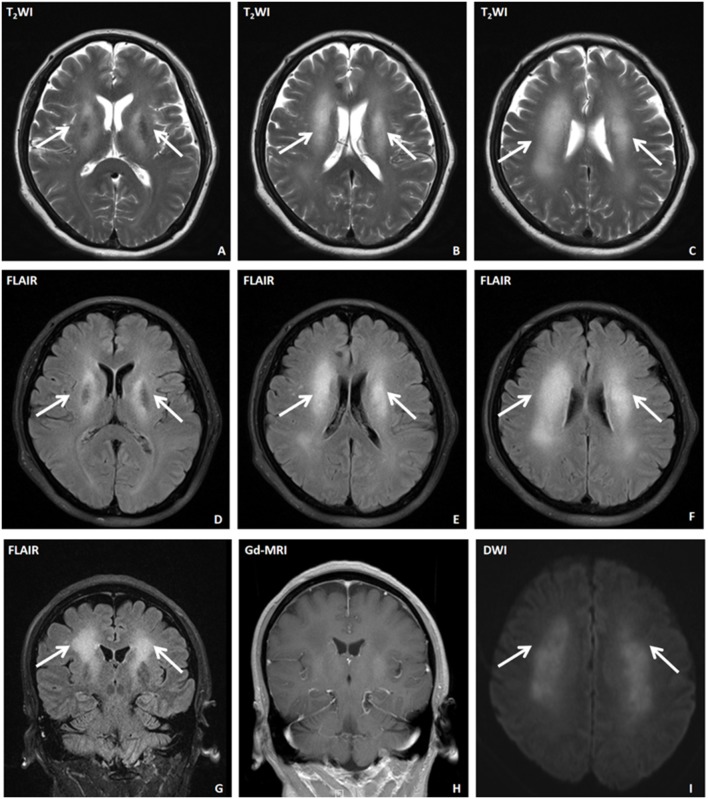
Cranial magnetic resonance imaging (MRI) of a patient with anti-CV2 autoimmune encephalitis. (A–C) T_2_WI axial images. (D–F) FLAIR axial images. (G) FLAIR coronal scan. (H) Enhanced MRI. (I) Diffusion-weighted MRI. The arrows indicate abnormal signals in the white matter area of the bilateral lateral ventricles and bilateral internal capsules. No significant enhancement was observed.
